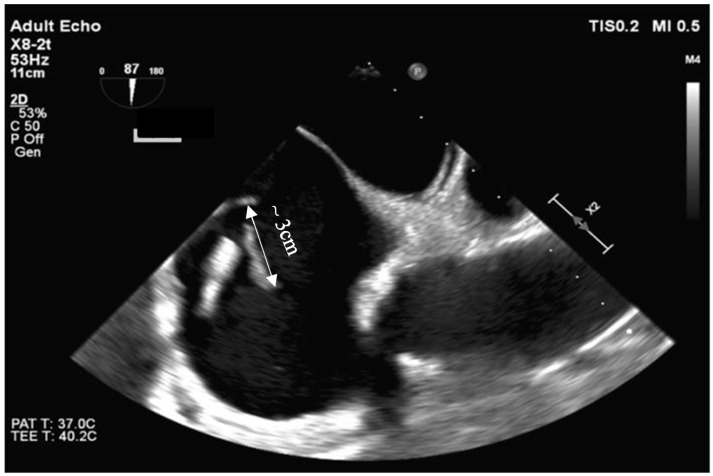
Transesophageal echocardiography demonstrating a ~3 cm vegetation on the ICD lead.
